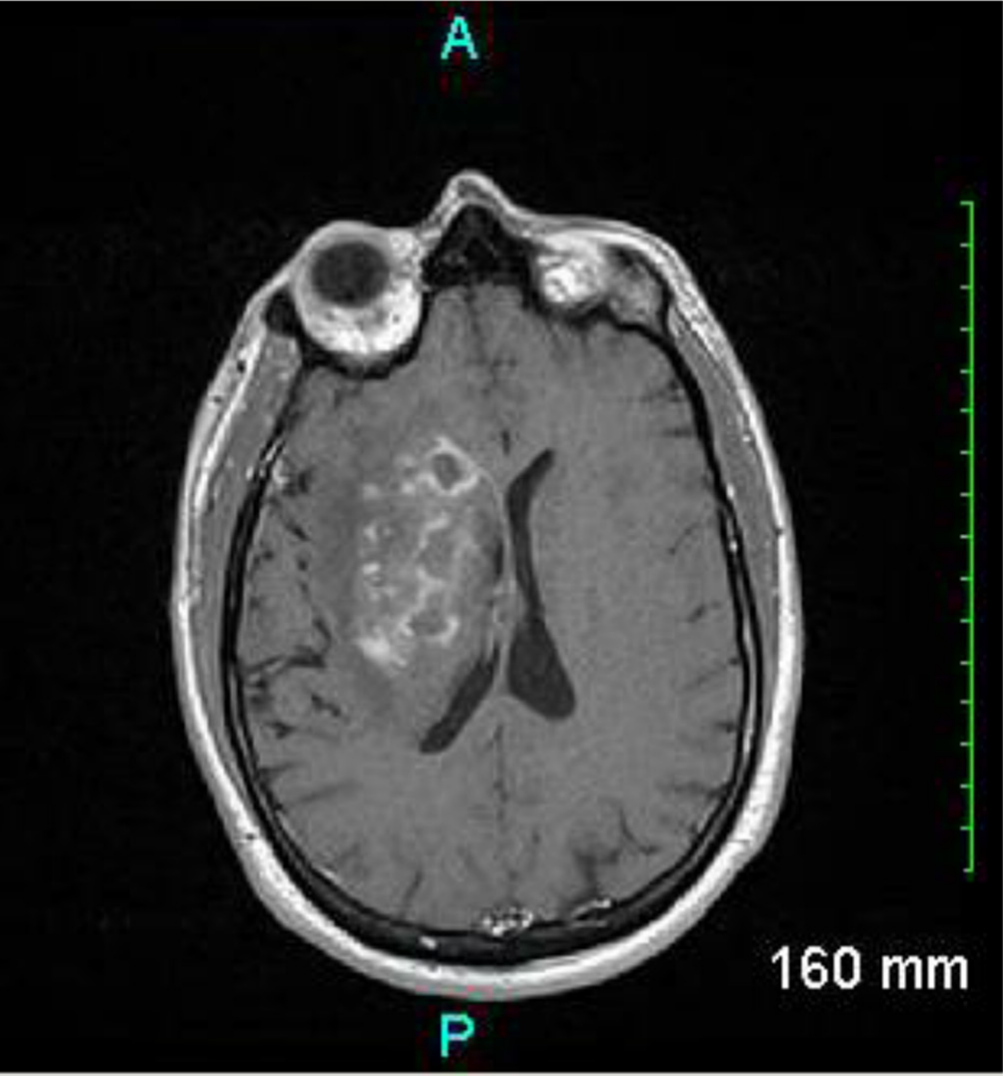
MRI of the brain with and without Omniscan IV contrast showing a heterogeneous enhancing lesion within the right basal ganglia with associated edema and local mass effect.
